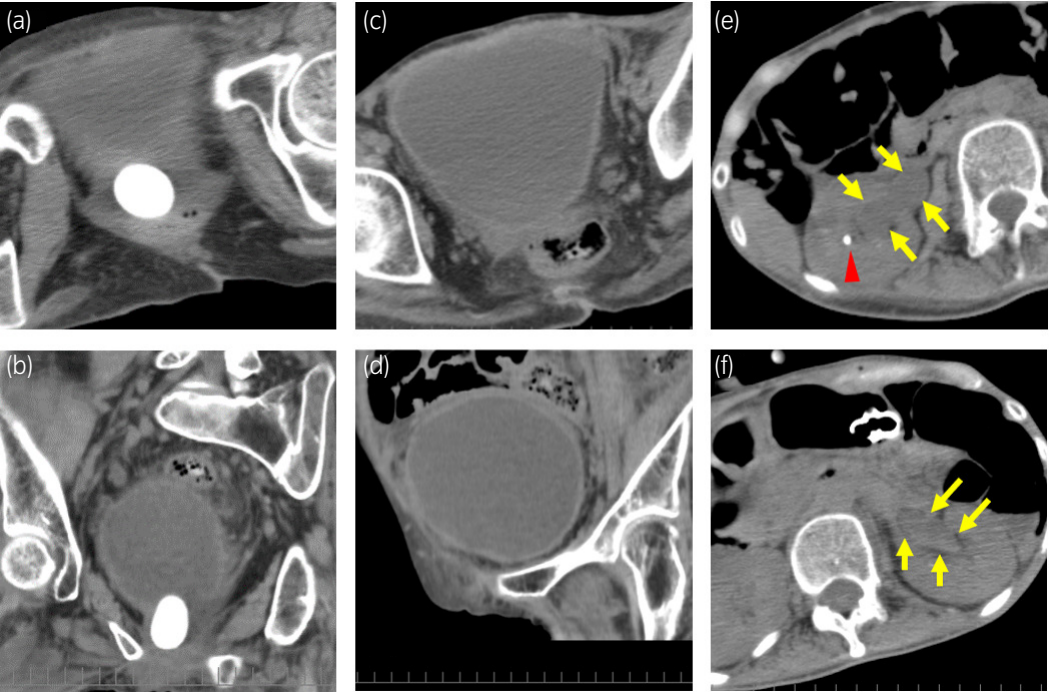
CT performed on the first day of hospitalization. (a,b) The giant calculus with a diameter of 3 cm was located on the dorsal and caudal side of the bladder. (c,d) A distended bladder was observed, and estimated volume of the bladder was about 690 mL (1/6 × 3.14 × 10 × 11 × 12). (e,f) The arrows showed bilateral hydronephrosis. The triangle indicated kidney stone.
